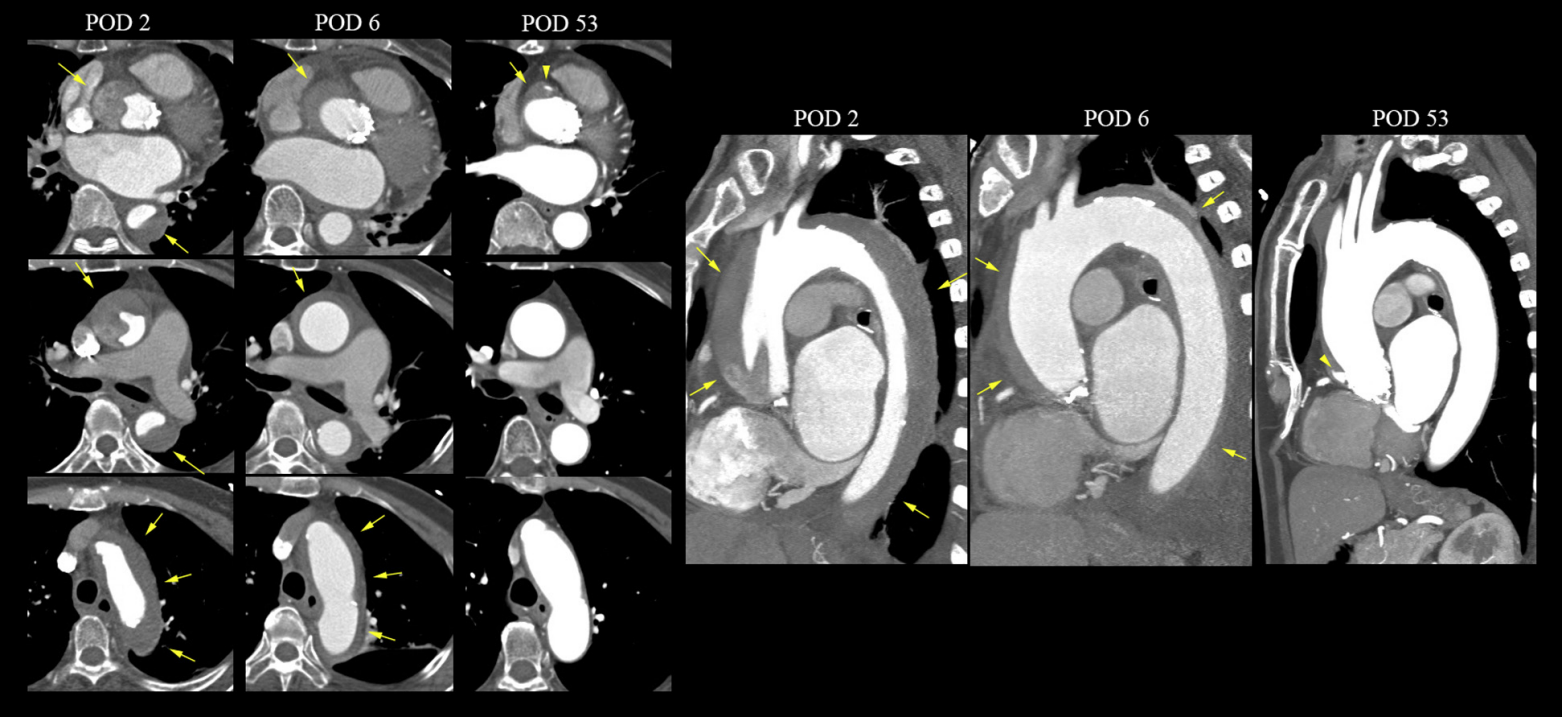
Serial Follow-Up Computed Tomography Images Serial follow-up computed tomography demonstrates the gradual resolution of the type A aortic dissection. Computed tomography on POD 2 reveals type A aortic dissection with the incompletely thrombosed false lumen (arrows) extending from the aortic root to the abdominal aorta. Computed tomography on postoperative day (POD) 53 shows the thrombosed false lumen resolution, leaving only a small focal outpouching contrast-filled lesion near the right coronary artery ostium (arrowheads).
